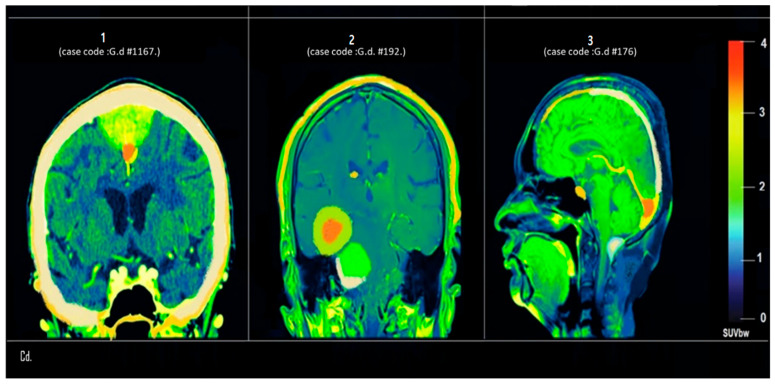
Coronal and sagittal PET/CT fusion images from Center D showing distinct radiotracer uptake patterns. Internal case codes (G.d-#1167, G.d-#192, G.d-#176) ensure data traceability. Color bar indicates SUVbw (standardized uptake value body weight). Panel 1 (G.d-#1167): intensely hypermetabolic midline suprasellar lesion (SUV >3.5), suggestive of hypothalamic–pituitary neoplasm. Panel 2 (G.d-#192): large right temporal lesion with central hypermetabolism (SUV ~4.0) and hypometabolic halo, characteristic of high-grade glioma. Panel 3 (G.d-#176): moderately hypermetabolic right posterior parietal–occipital lesion (SUV 2.5–3.0), indicative of primary or metastatic neoplasm with mass effect.
